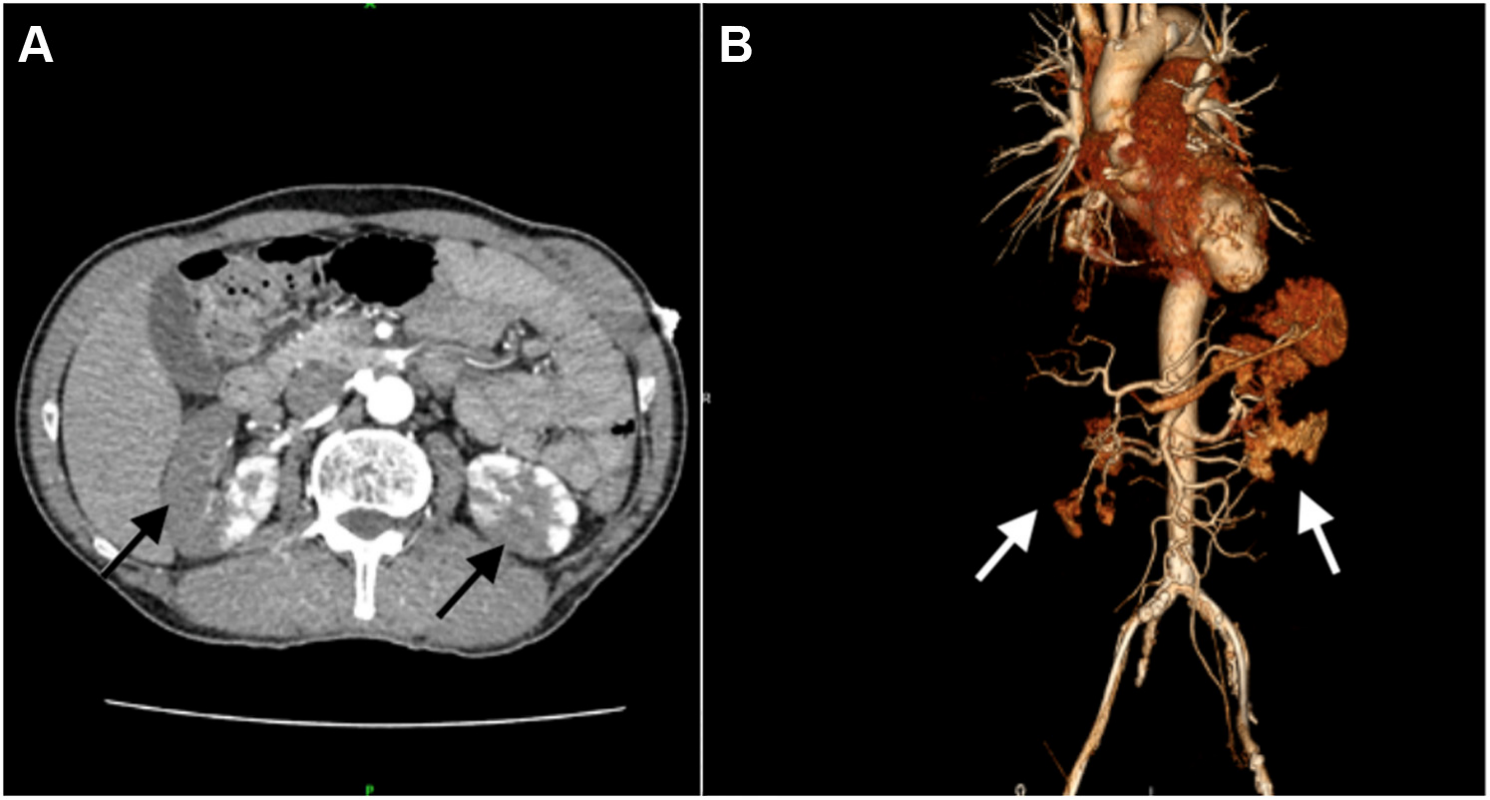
Renal Infarcts (A) Abdominal computed tomography scan with contrast enhancement revealed multiple extensive patchy infarcts in both kidneys (black arrows). (B) 3-dimensional reconstruction with barely visible of both renal tissue (white arrows).
